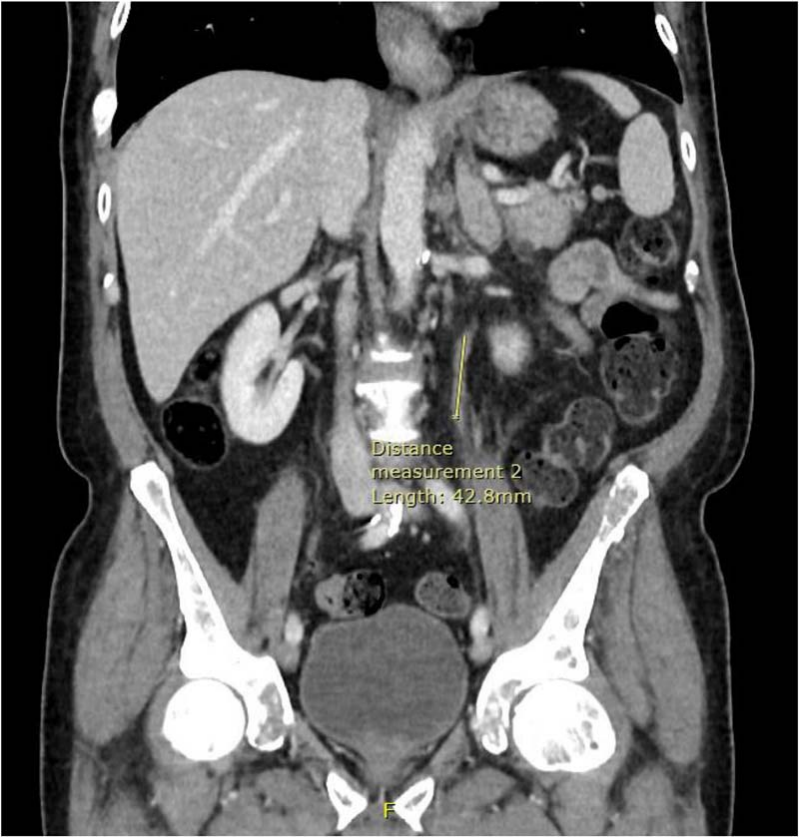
Coronal CT abdomen and pelvis scan with contrast demonstrating urethral enhancement for a distance of 42.8 mm.
